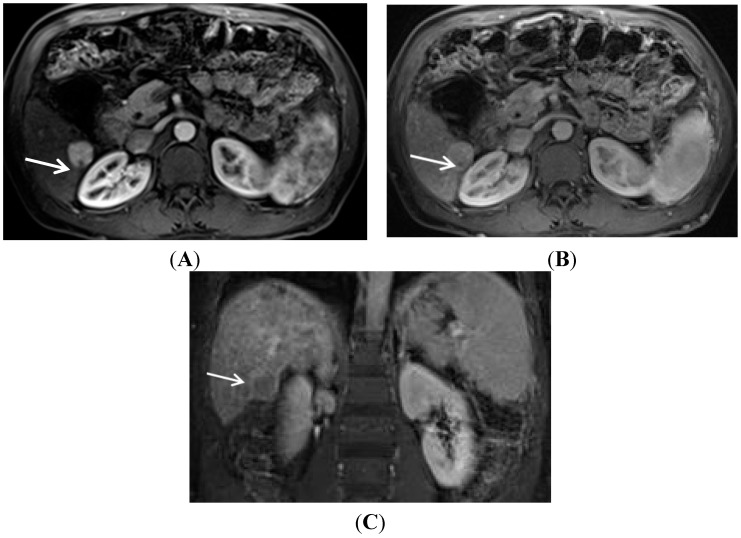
Typical contrast enhancement for MRI diagnosis of HCC. (A) On a late arterial phase image, a 2.3 cm HCC (arrow) demonstrates unequivocal arterial enhancement relative to the surrounding liver; (B) On the portal venous phase of the same exam, the hepatocellular carcinoma (arrow) has decreased in enhancement relative to the earlier arterial phase while the hepatic parenchyma has increased in signal intensity; (C) A coronal image obtained in the equilibrium phase demonstrates the hepatocellular carcinoma (arrow) as being of lower signal intensity than the adjacent cirrhotic parenchyma (“washout”). Additionally present is the delayed capsule or so-called pseudo-capsule, which helps further solidify the imaging diagnosis of hepatocellular carcinoma.
